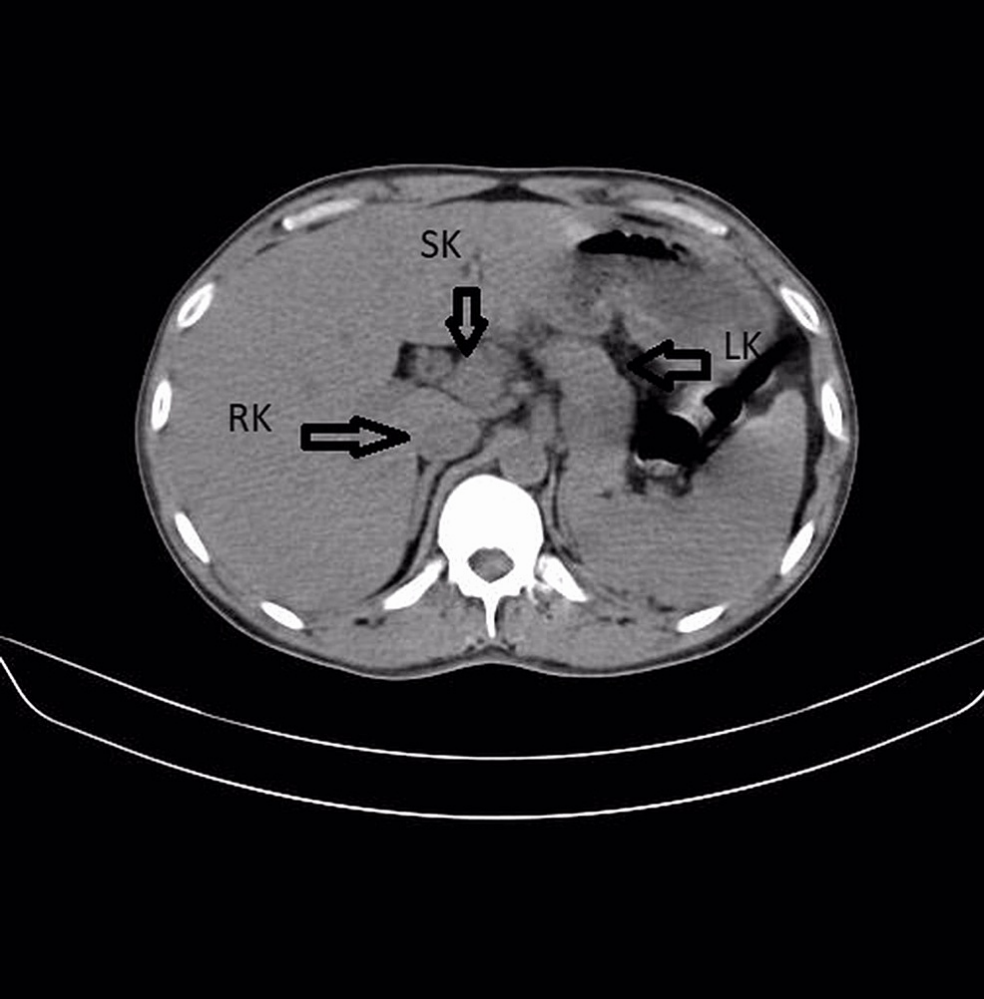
Abdominal CT scan axial image shows fusion of right kidney and supernumerary kidney anteriorly at lower poles
